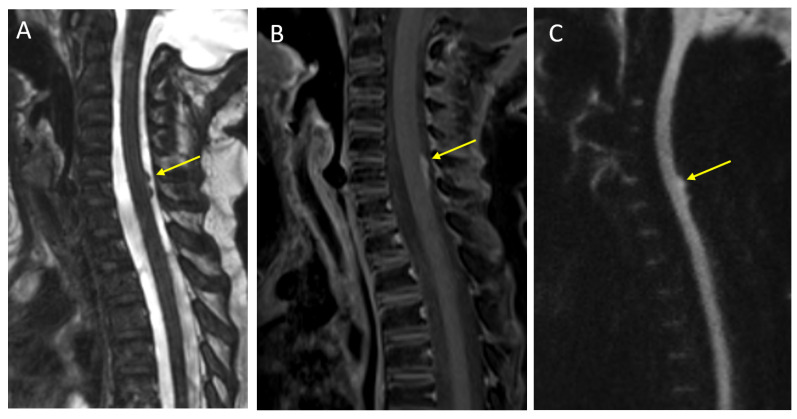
A 6-year-old girl with leptomeningeal metastasis from medulloblastoma. Sagittal CISS (A) and post-contrast T1 (B) imaging demonstrate nodular and enhancing metastatic foci along the dorsal cervical spinal cord (arrows). DWI (C) imaging shows associated diffusion restriction.
